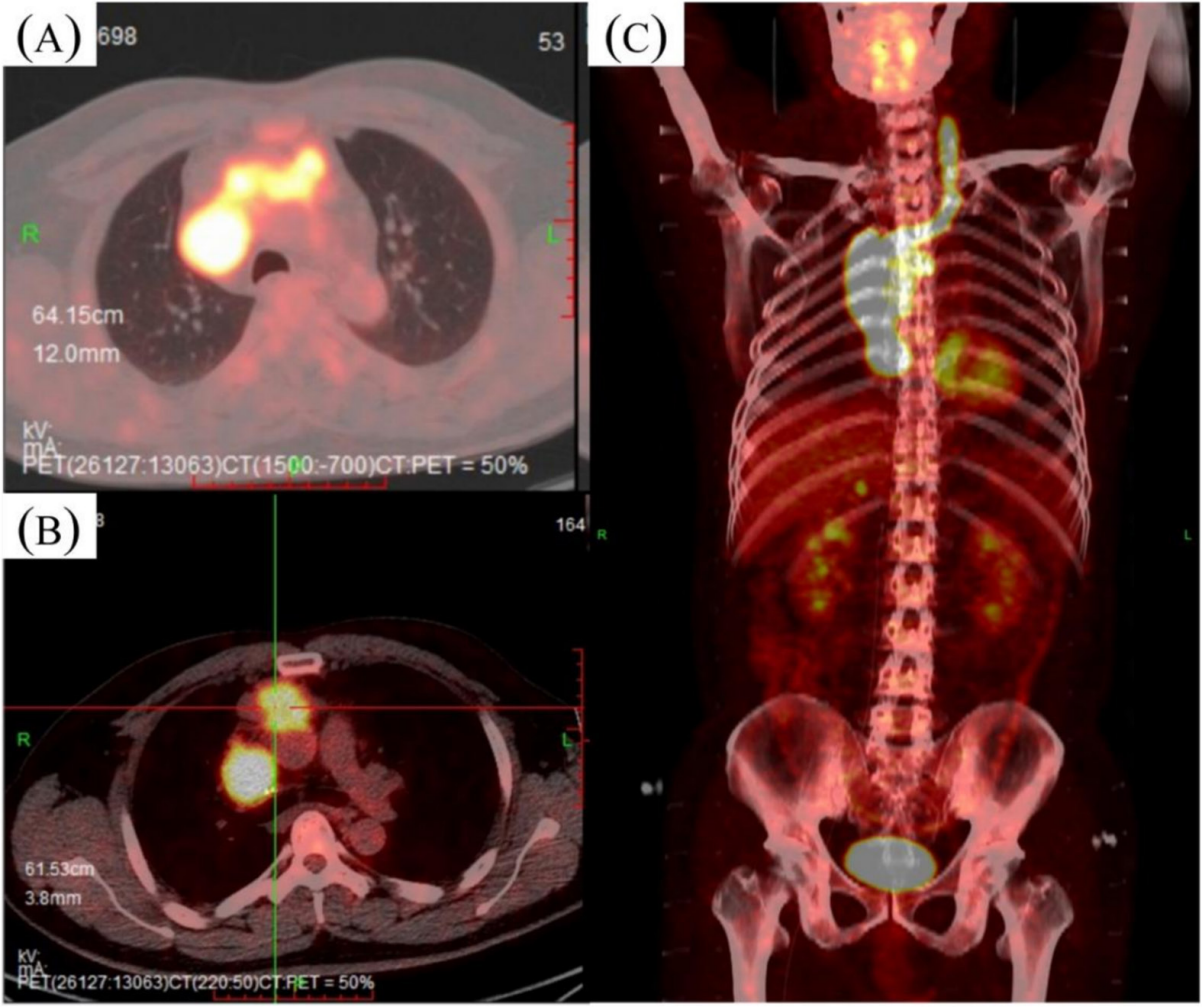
PET‐CT images of the case. (A,B) Images showing thoracic mediastinal window treatment. (C) Local PET‐CT images of the coronal plane.
